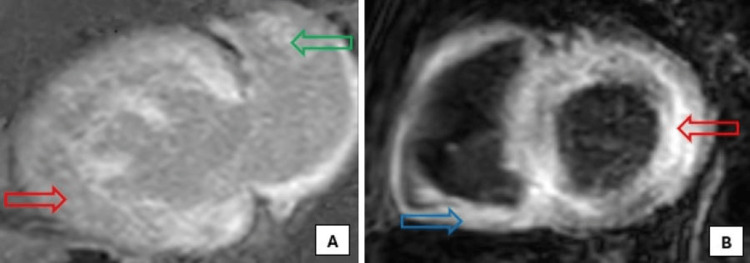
Cardiac magnetic resonance imaging showing global and subendocardial left ventricle (red arrows), right ventricle (blue arrow), and left atrium (green arrow), myocardial fibrosis on delayed gadolinium enhancement due to abnormal contrast kinetics, consistent with cardiac amyloidosis (A - two-chamber view, B - short-axis view).
